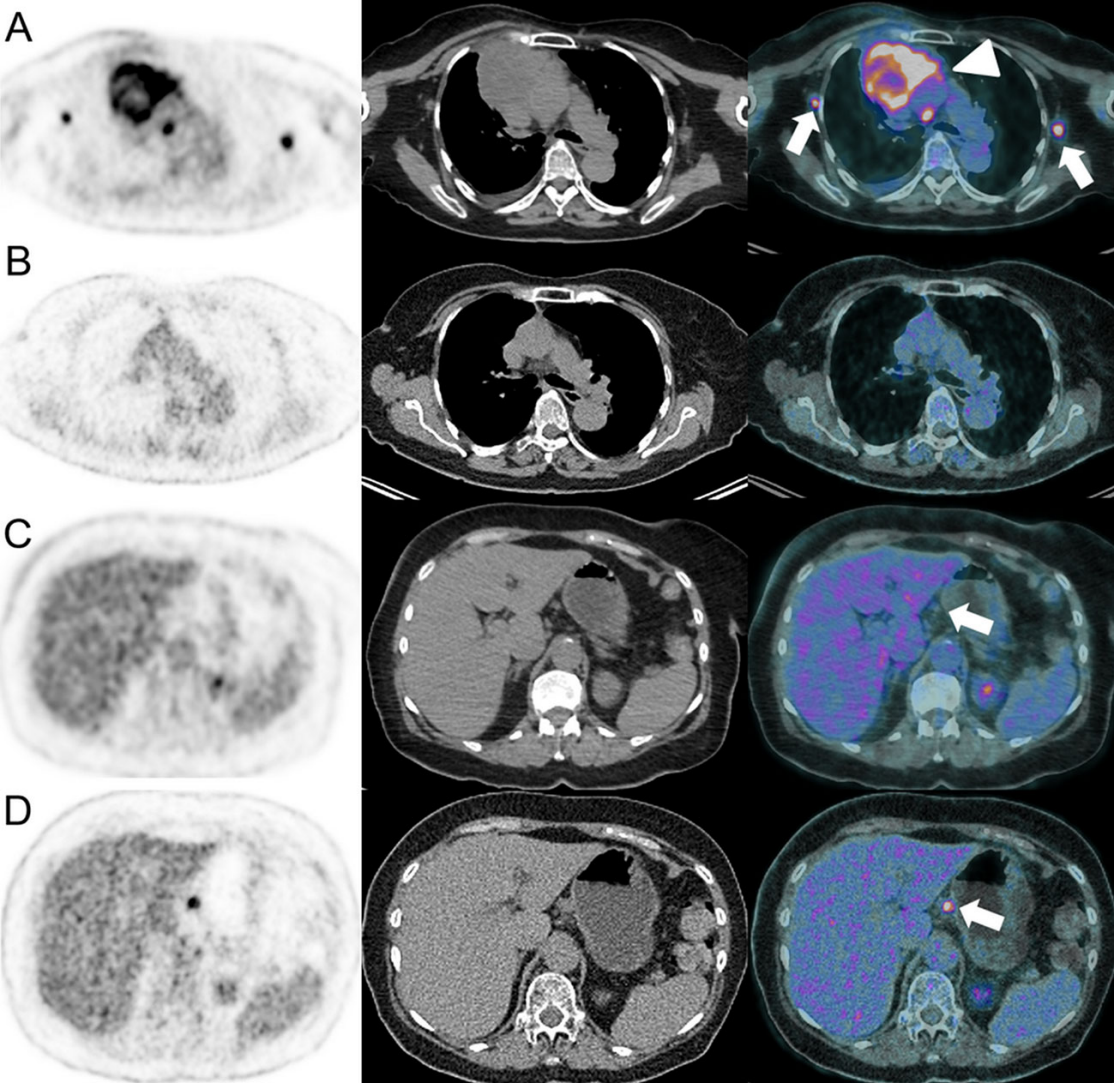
Dissociated response in a 63-year-old female with left lower leg Meckel carcinoma and mediastinal [(A), arrowhead] and axillar [(A), arrows] metastatic spread as demonstrated on baseline ^18^F-FDG-PET/CT (A). Pembrolizumab (anti-PD-1) was administered. At the 6-months follow-up, ^18^F-FDG-PET/CT showed complete response on the mediastinal and axillar lymph nodes (B). However, a hypermetabolic coeliac lymph node appeared [(D), arrow], which was not present at baseline [(C), arrow].
